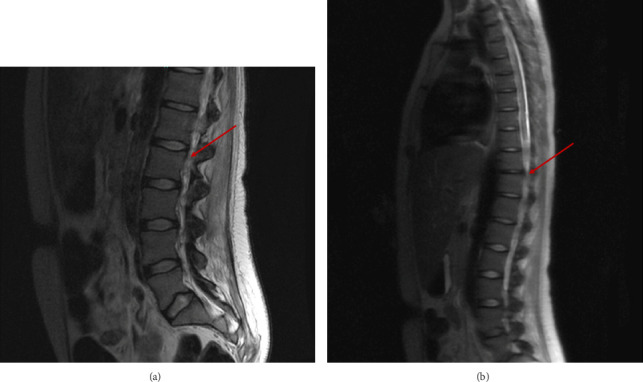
(a) Repeat MRI of the lumbar spine demonstrating diffuse spinal cord swelling with central T2 bright signal and peripheral enhancement. (b) Repeat MRI of the thoracic-lumbar spine demonstrating diffuse spinal cord swelling with central T2 bright signal and mild diffuse atrophy of the thoracic spinal cord—particularly in the mid-to-lower thoracic segments.
